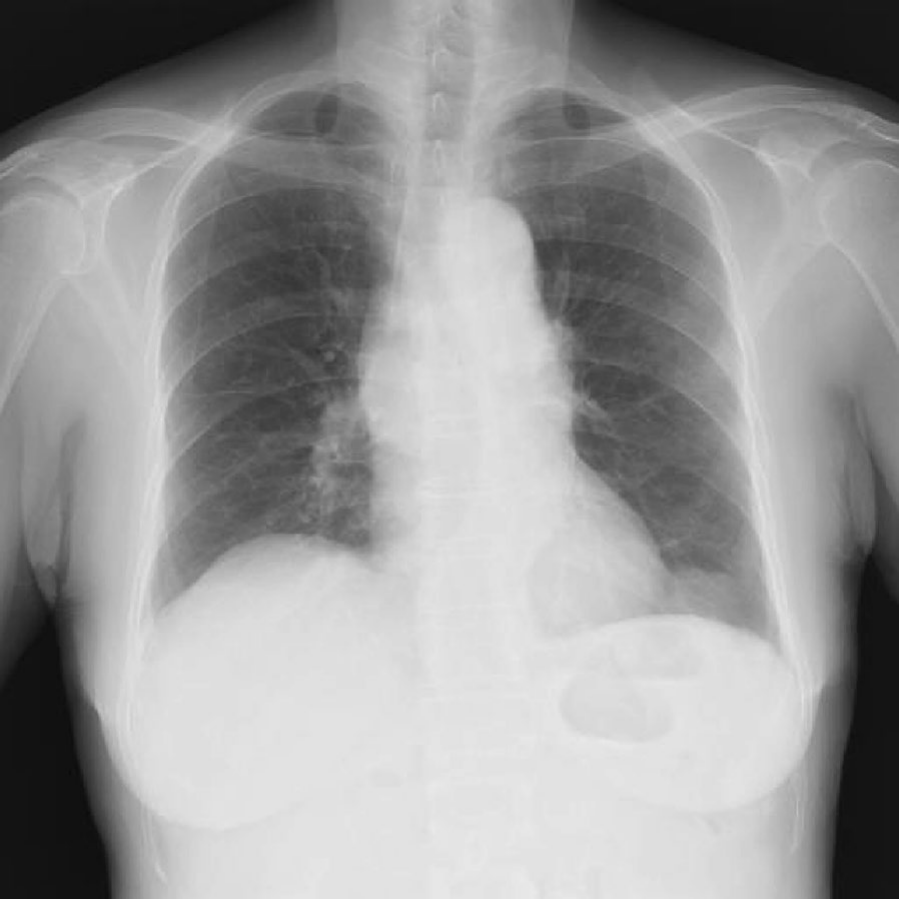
Chest X-ray showed no abnormal findings.
